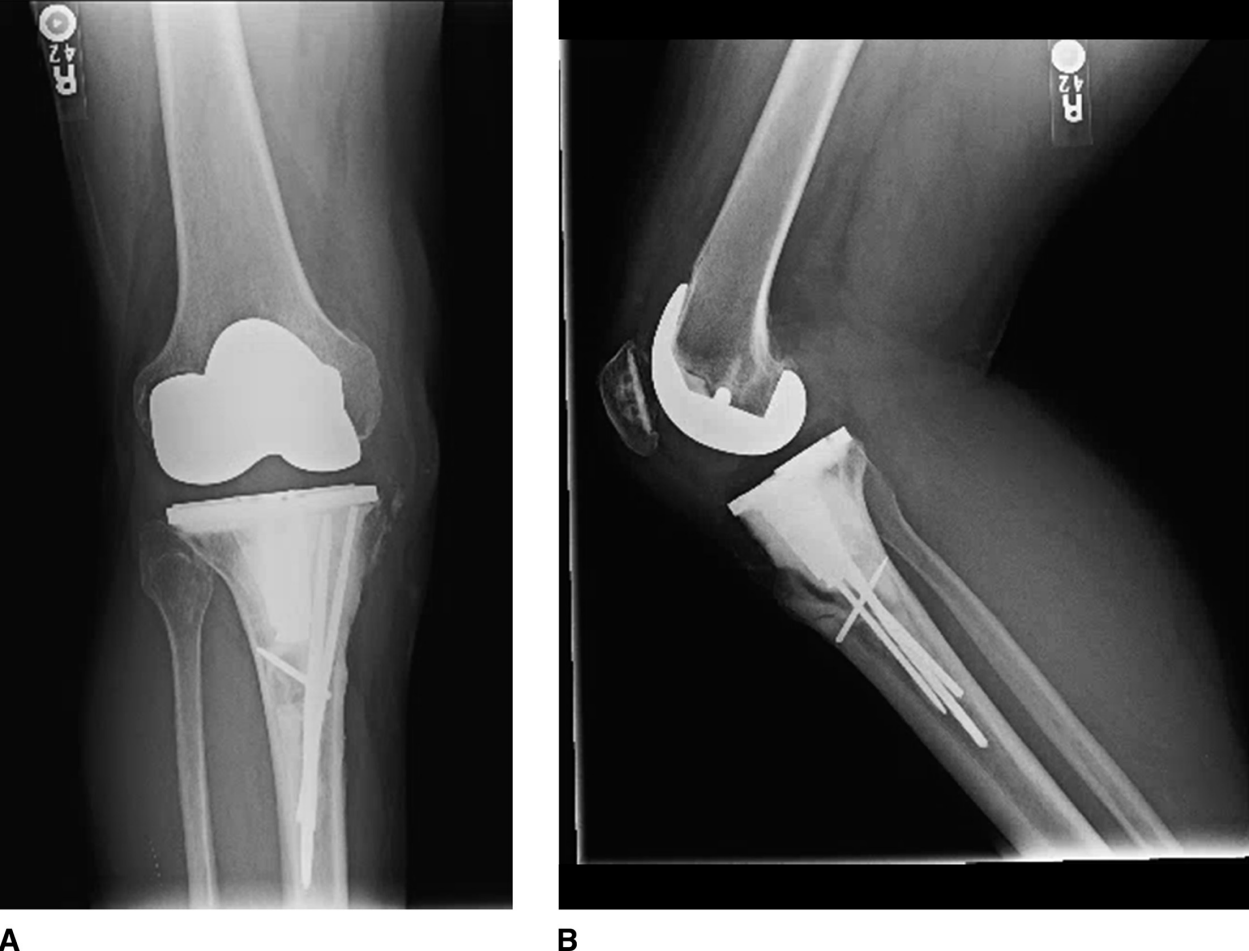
AP (A) and lateral (B) 16-month postoperative radiographs demonstrating maintained alignment without evidence of component subsidence or implant failure.
